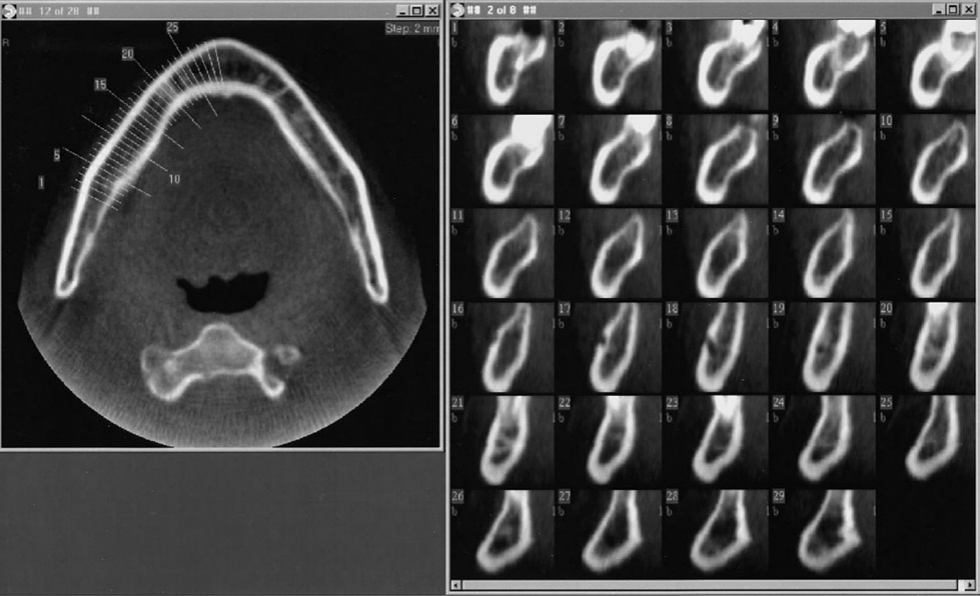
First cone beam CT for clinical application in dentistry. *Note*. Reprinted from “A new volumetric CT machine for dental imaging based on the cone-beam technique: preliminary results”, by Mozzo P, et al., *Eur. Radiol* 1998; 8:1560, Figure 4.
